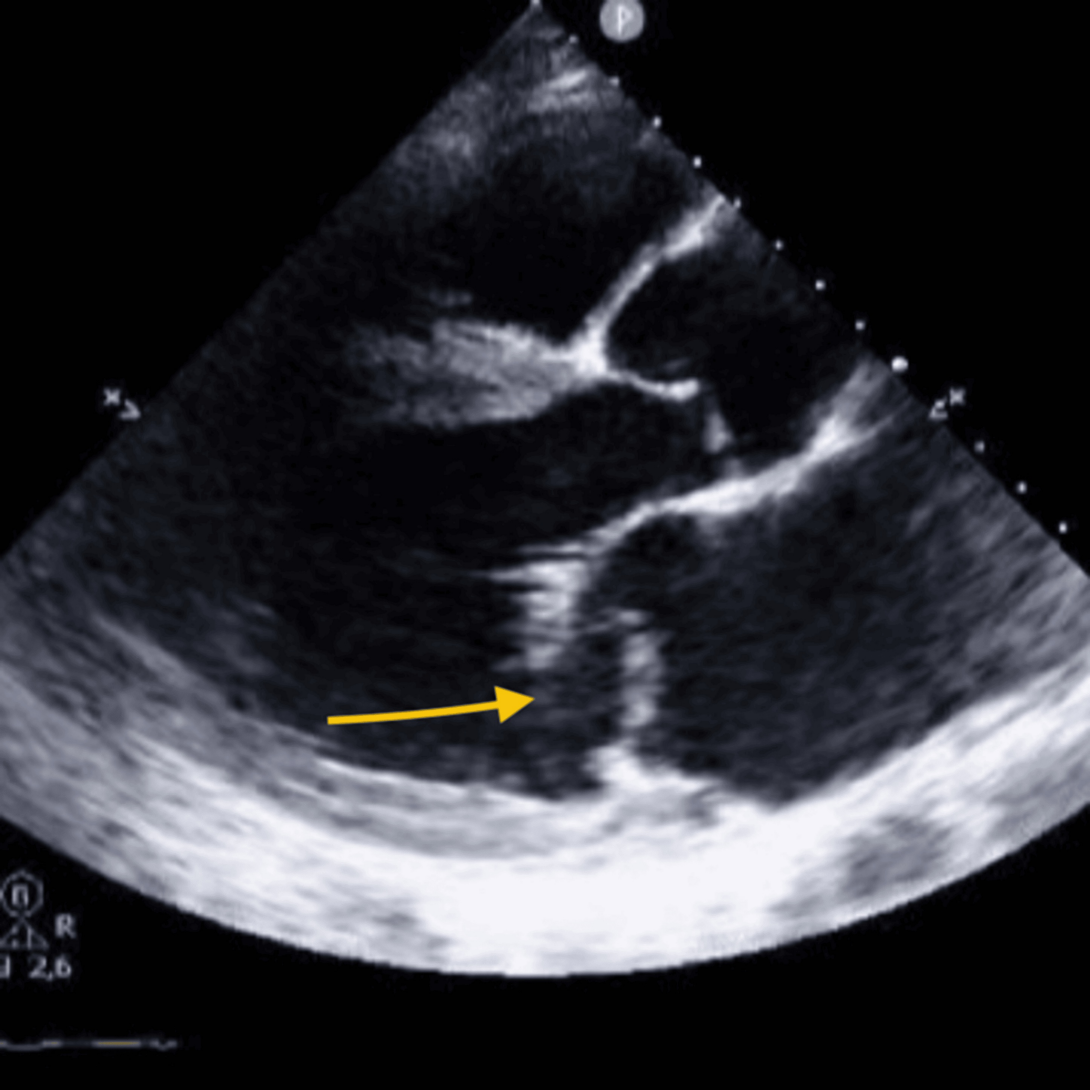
Transthoracic echocardiogram parasternal long-axis view revealing a major mitral regurgitation
